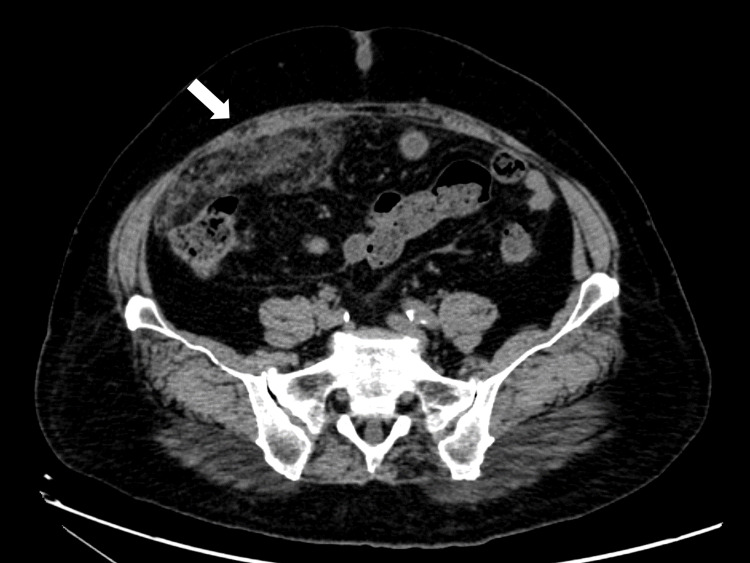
Follow-up CT. The image shows enhancement of the right lower peritoneum mass. CT: computed tomography
